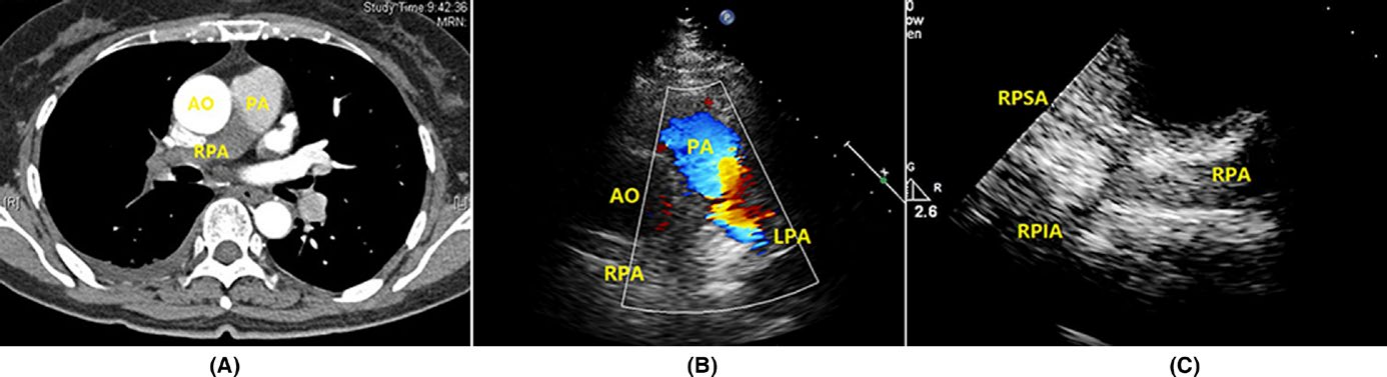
A. CTPA showed filling defects and luminal occlusion in right pulmonary artery. B. Parasternal aortic short‐axis view showed right pulmonary artery filling defects by CDFI. C. Suprasternal right pulmonary artery long‐axis view showed luminal medium echogenic signals and luminal occlusion in right pulmonary artery (movie clips S4 and S5). AO = aorta; PA = pulmonary artery; RPSA = right pulmonary superior artery; RPIA = right pulmonary inferior artery; LPA = left pulmonary artery; RPA = right pulmonary artery; CDFI = color Doppler flow imaging; CTPA = computed tomographic pulmonary angiography
